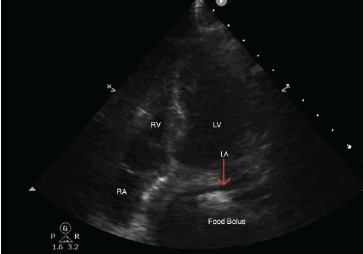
Apical 4-chamber cardiac view from point-of-care ultrasound showing a food bolus causing gastric outlet obstruction and external compression of the left atrium (arrow). *RA*, right atrium; *RV*, right ventricle; *LV*, left ventricle; *LA*, left atrium.
